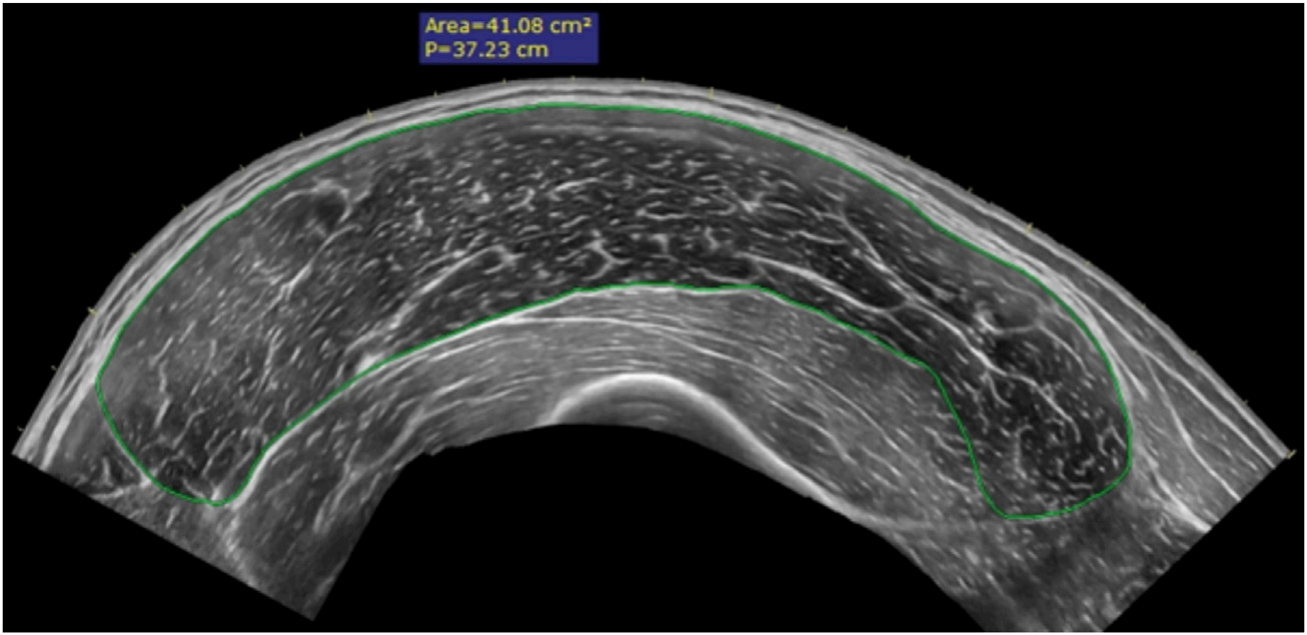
A typical panoramic ultrasound image is used to measure the anatomical cross-sectional area (ACSA) within the fascia of skeletal muscles, indicated by the green line.
